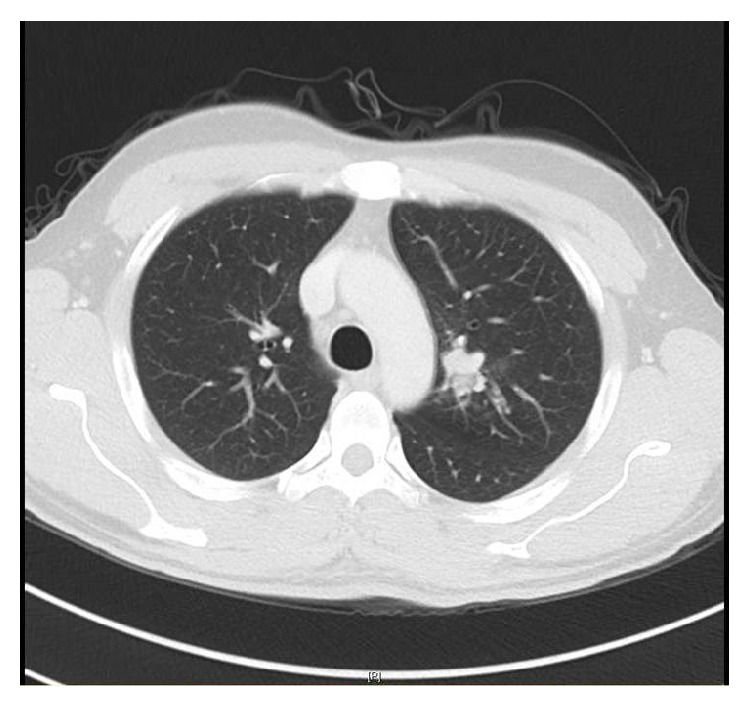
The Computed Tomography (CT) scan showed a lobulated round nodule in the left upper lobe adjacent to the bronchus measuring 1.8 × 1.6 cm with an absence of significant lymph nodes. It is associated with a focal area of ground glass opacity and mild dilatation with mucus plugging.
